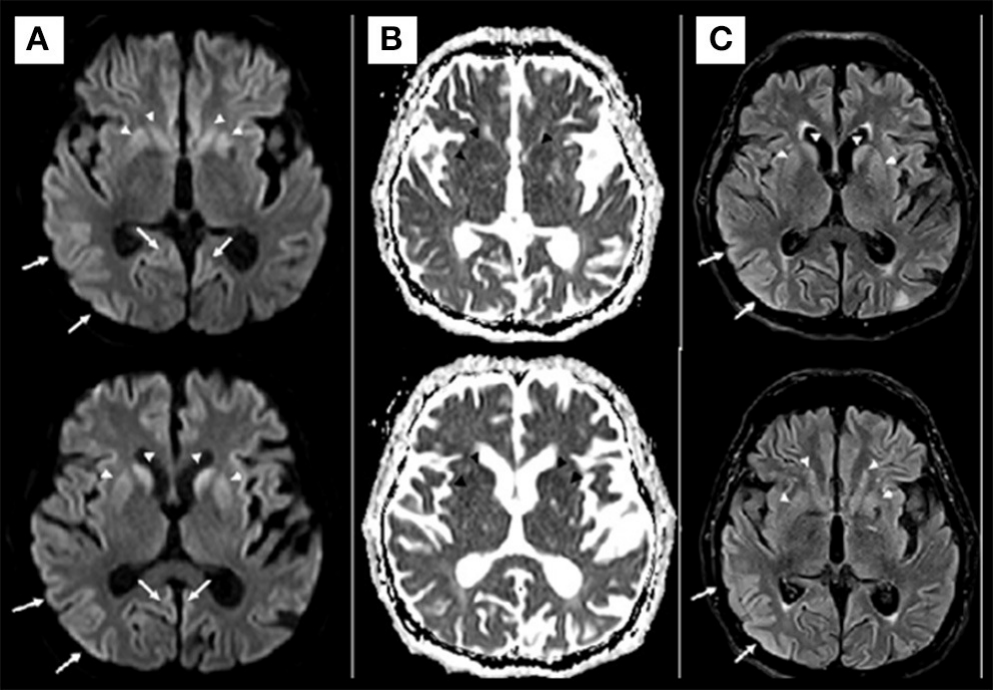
Axial diffusion [diffusion weighted imaging (DWI)] (A) and apparent diffusion coefficient (ADC) map (B) MRI at admission. Diffusion restriction involving the cerebral cortex and basal ganglia (arrows in A). No convincing white matter involvement. The hypotheses for MRI findings were pos-ictal state, hypoxic-ischemic encephalopathy, and spongiform encephalopathy. Axial Diffusion and FLAIR (C) MRI after 2 weeks demonstrated similar imaging findings, making the hypothesis of spongiform encephalopathy more likely.
